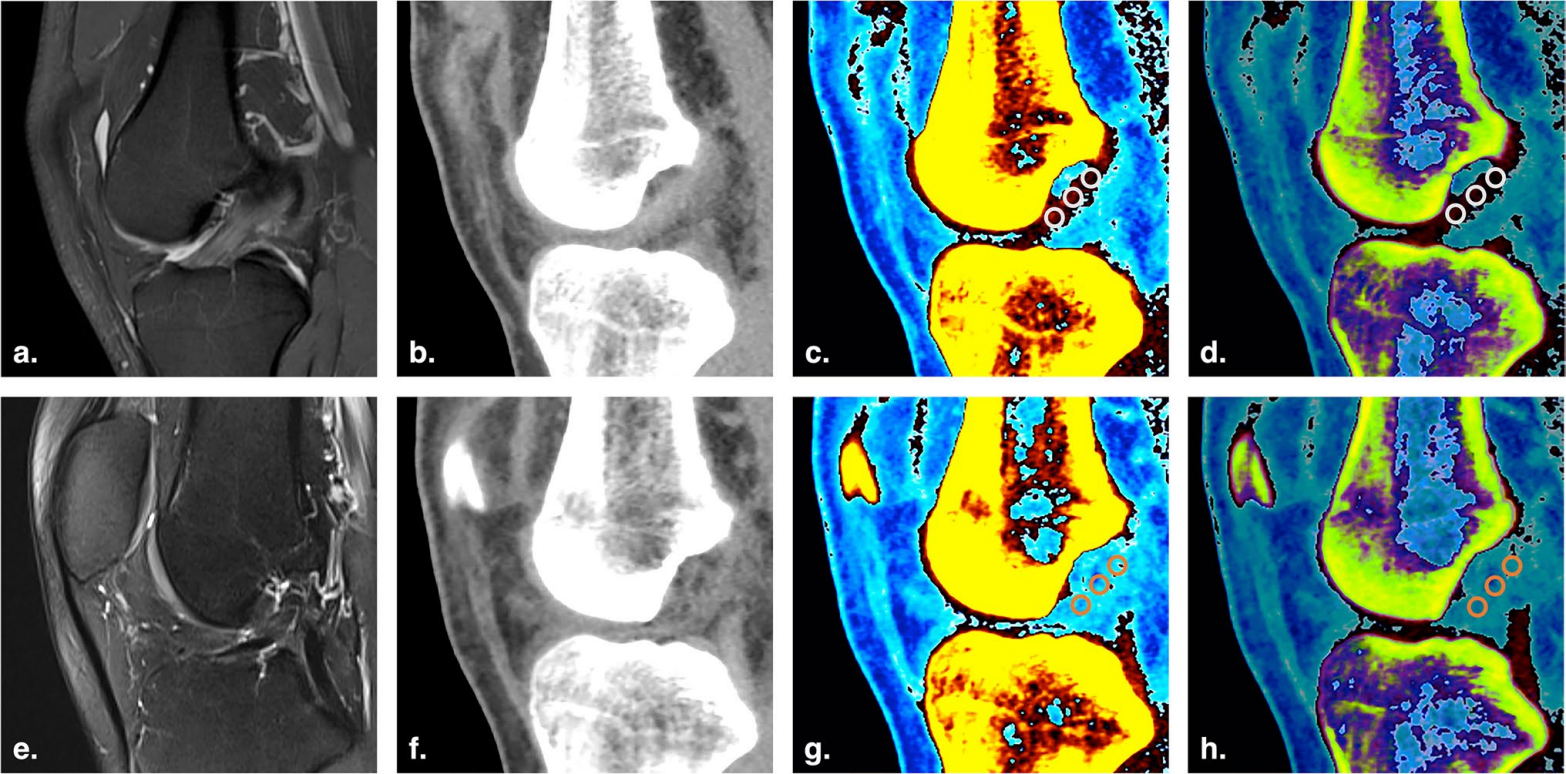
Images of a 25-year-old man with left ACL rupture. a and e Sagittal proton density-weighted and T2-weighted fat-saturated MRI images of the right and left knees, respectively. b and f Oblique sagittal plane, monochromatic DECT images with normal mode (mixed keV) of the right and left knees, respectively. c and g Oblique sagittal plane, color-coded DECT images with mono + mode (80 keV) of the right and left knees, respectively. d and h Oblique sagittal plane, color-coded DECT image with Rho/Z mode (mixed keV) of the right and left knees, respectively. The intact ACL was colored black and dark red in the anatomic position (c and d). The torn ACL was not colored (g and h). Three circular ROIs were set on the proximal, middle, and distal sites of the normal ACL (c and d) and torn ACL (g and h), respectively
